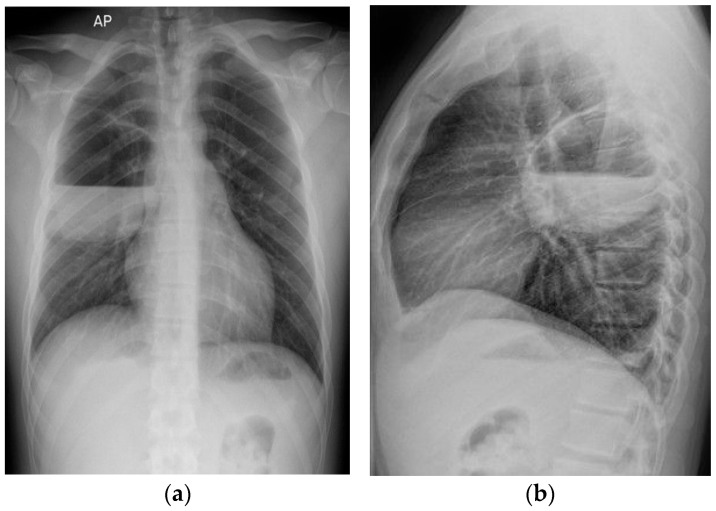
Chest-X-ray evaluation 5 days after admission shows an air-fluid image located in the right middle lobe, with 86/95/90 mm (anteroposterior/transverse/craniocaudal), well delimited with slightly irregular contour, occupied in the lower half by fluid level. (a) Antero-posterior (AP) chest-X-ray. (b) Profile-view chest X-ray.
